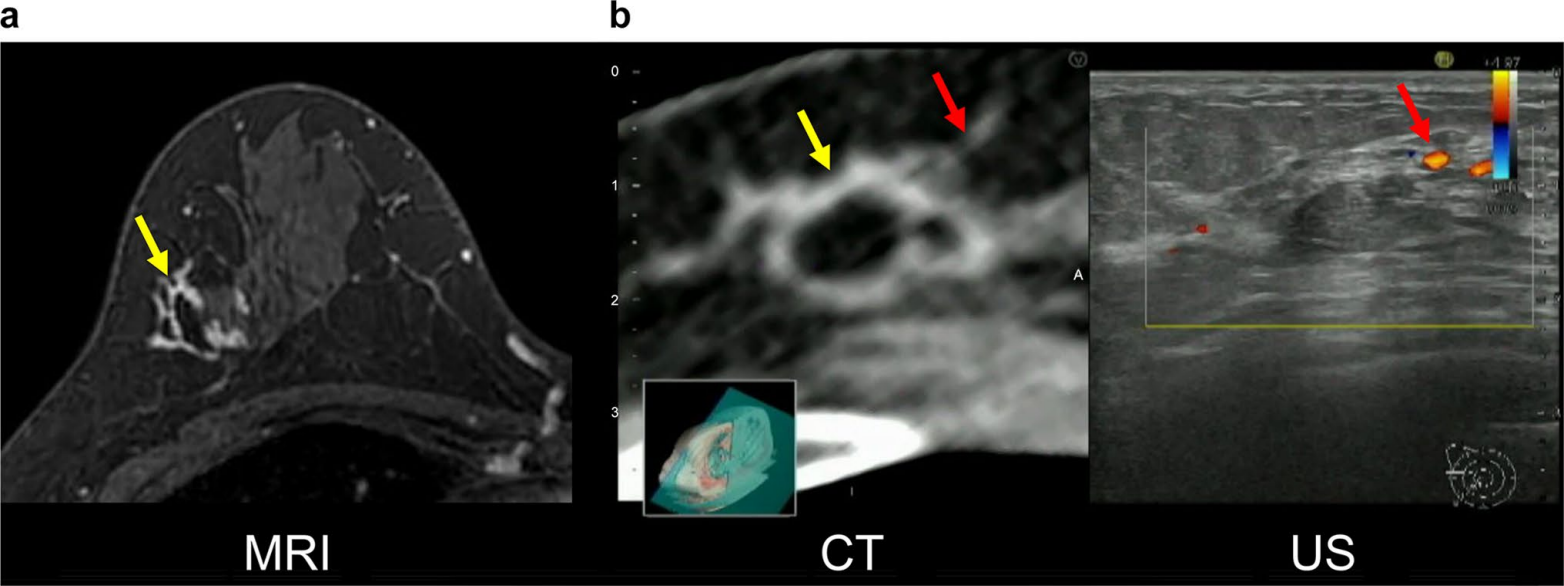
CT/ultrasound fusion image of non-mass enhancement. a Non-mass enhancement (yellow arrow) can be seen spreading regionally under MRI. b After confirming the region corresponding to non-mass enhancement visualized on the prone position MR image on the contrast-enhanced CT image (yellow arrow), fusion of CT and ultrasound using RVS^®^ was performed. When fusing the images of different modalities, it is important to align the positions of the fat around the lesion, the shape of the mammary gland and ribs, and the blood vessels (red arrow), and to visualize the same cross-section
